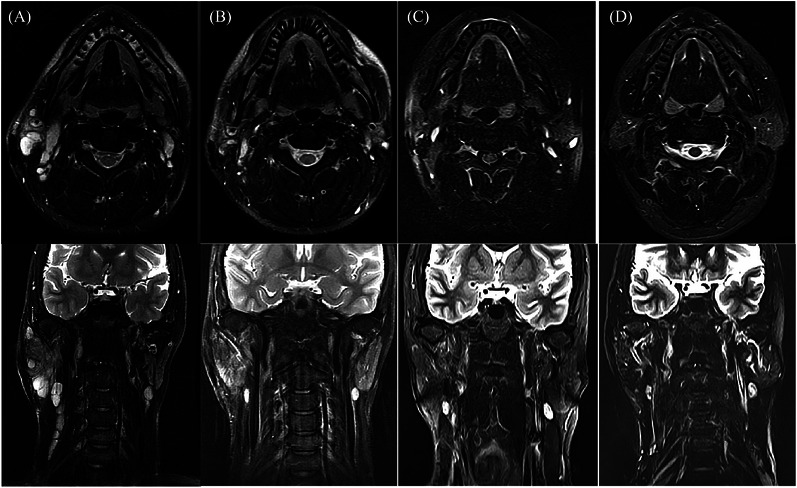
T2 magnetic resonance images of patient KD 07 (19 year‐old man). (A) Before, (B)1 month after, (C) 2 years after, and (D) 14 years after radiotherapy.
